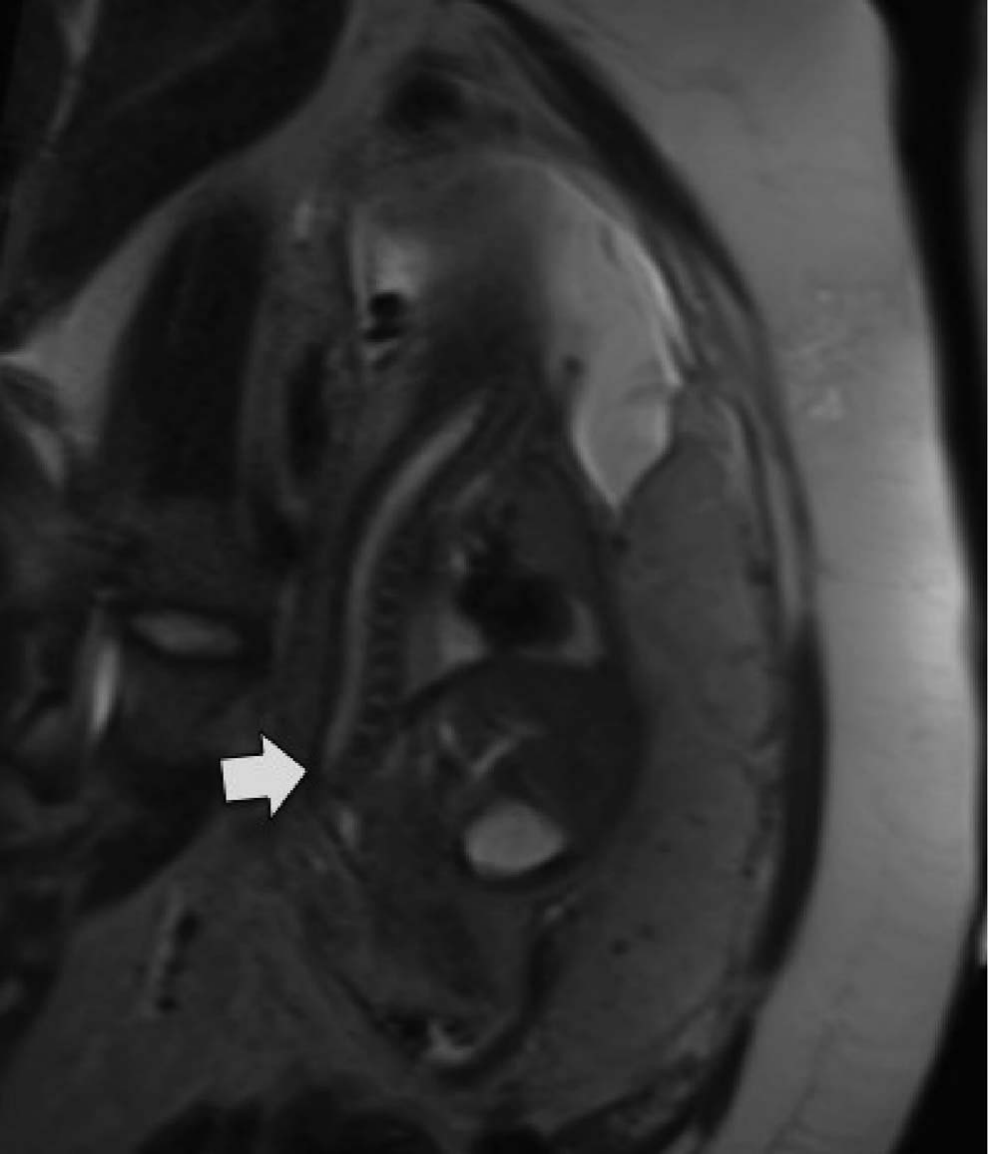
Fetal magnetic resonance imaging. T2-weighted HASTE (half-Fourier single-shot turbo spin-echo) oblique reconstruction in the sagittal plane of the thoracolumbar and sacral spine shows a decreased number of lumbar vertebrae and a diminutive sacrum (arrow).
